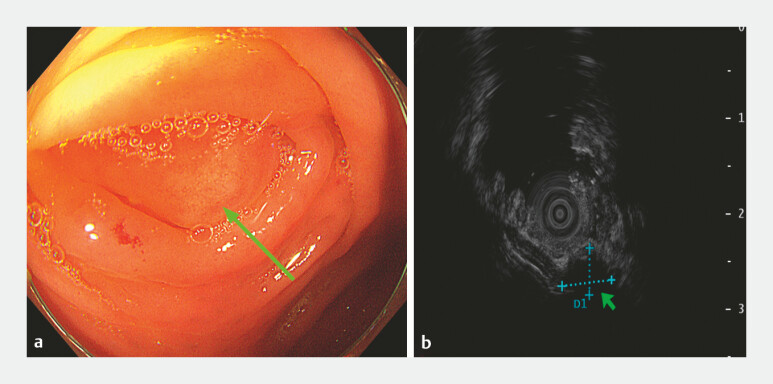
Endoscopic and endosonographic appearance of the lesion (green arrow).
